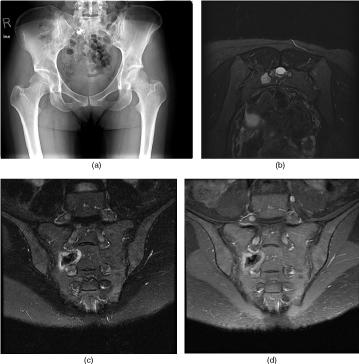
Imaging appearance after microwave ablation and cementoplasty. AP radiograph of the pelvis (a) shows ablation and cementoplasty-related changes. MRI of the pelvis with axial and coronal *T*_2_ weighted (b, c; FSE FS, TR = 3000 ms, TE = 102 ms, 512 × 512, 4-mm thickness) and post-contrast *T*_1_ weighted images (d; FSE FS + C, TR = 517 ms, TE = 11 ms, 512 × 512, 4-mm thickness) demonstrates a central hypointensity with peripheral *T*_2_ hyperintense rim and homogeneous rim of enhancement. There was also irregularity of the right S3 nerve root related to treatment changes. AP, anteroposterior; FSE FS, fast spin echo, fat saturated; FSE FS + C, post-contrast fast spin echo, fat saturated; TE, echo time; TR, repetition time.
